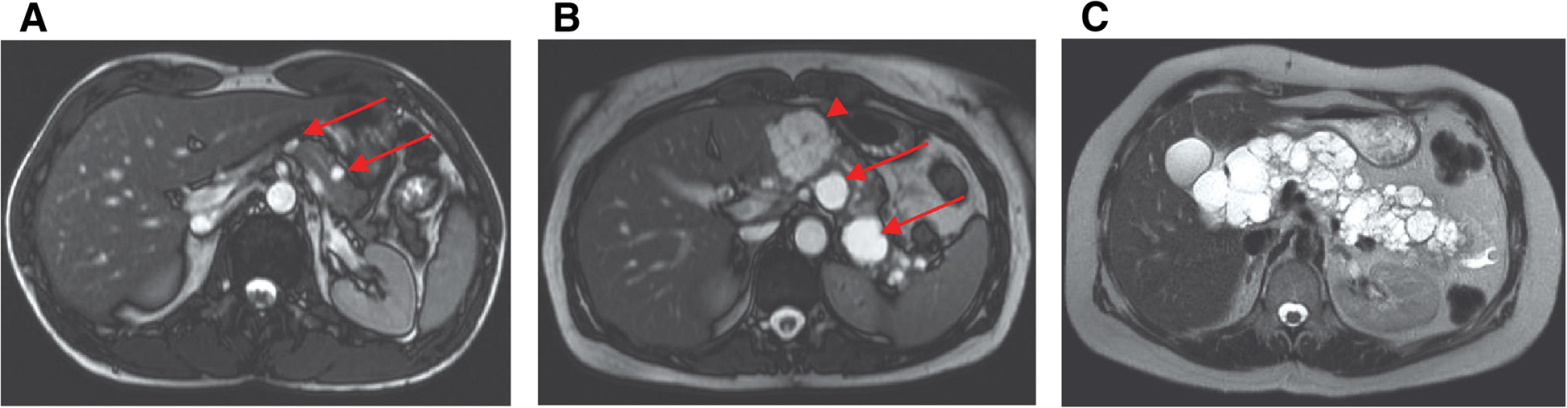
Axial MRI images of pancreatic involvement in three VHL patients. None of these patients had pancreas-related symptoms or exocrine/endocrine insufficiency. (A) Simple cysts (arrows) with size <1 cm in a 32 year old man; (B) A 4 cm sized serous microcystic cystadenoma (arrowhead) is present next to multiple simple cysts (arrows) in a 39 year old woman; (C) Shows replacement of almost the entire pancreas by multiple cysts in a 47 year old woman.
